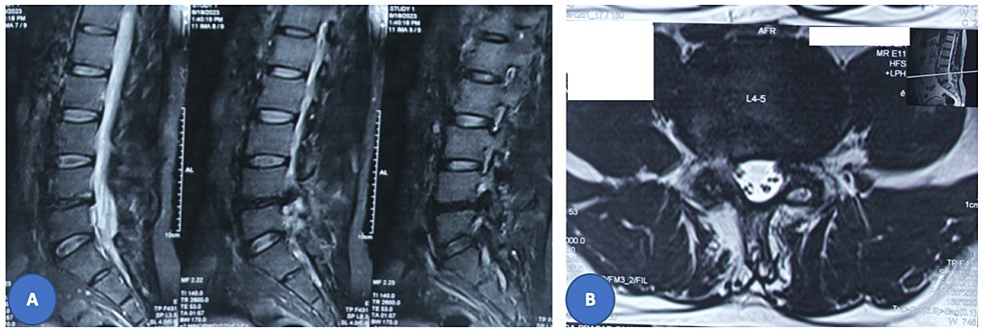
Preoperative MRI images of revision surgery showing the L4-5 level laminectomy defect and left paracentral disc extrusion contiguous with the posterior disc margin suggestive of recurrent herniated disc. (A) sequential T2 images of the sagittal section; (B) axial images at the L4-5 level.
